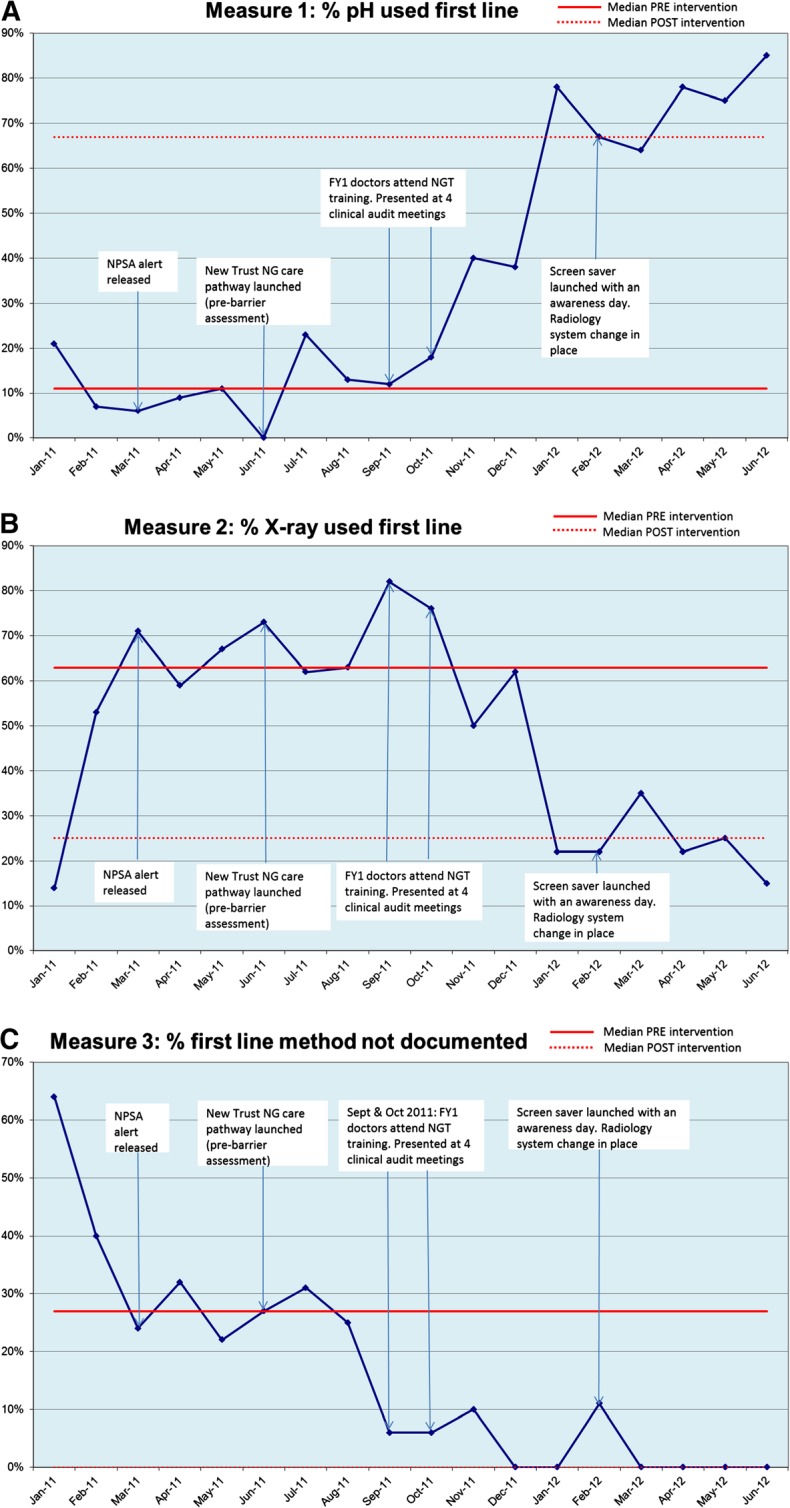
Time trend for use of pH as first line method used to check tube position. 1A: Use of pH as first line method to check tube position. 1B: Use of X-ray as first line method to check tube position. 1C: First line method to check tube position not documented.
